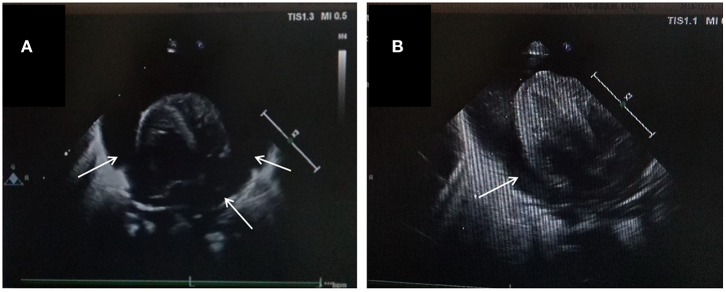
Echocardiographic image in the apical four chamber view shows a large pleural effusion. Pericardial cavity fluid dark area were detected: 6.2 mm in the rear of posterior wall of left ventricle, 6.5 mm in front of anterior wall of right ventricle, 9.1 mm in front of apical part, 5.6 mm outside the lateral wall of left ventricle, 9.8mm outside the lateral wall of right ventricle, 6.3 mm under the inferior wall of right ventricle beneath xiphoid process. Light spot reflex were visible in pericardial cavity. Left ventricular ejection fraction was 53%. (A) Apex four-chamber view of the heart. (B) Parasternal four-chamber view of the heart.
